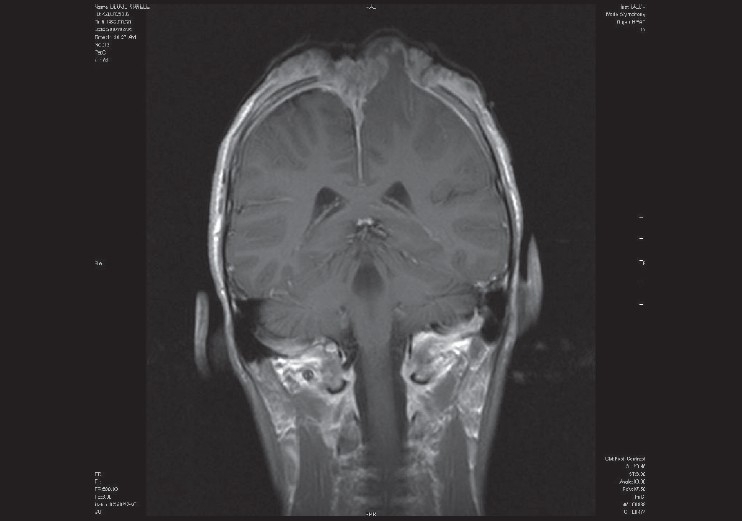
MRI scan showing tumour infiltration of the left cerebral hemisphere and the falx celebri
